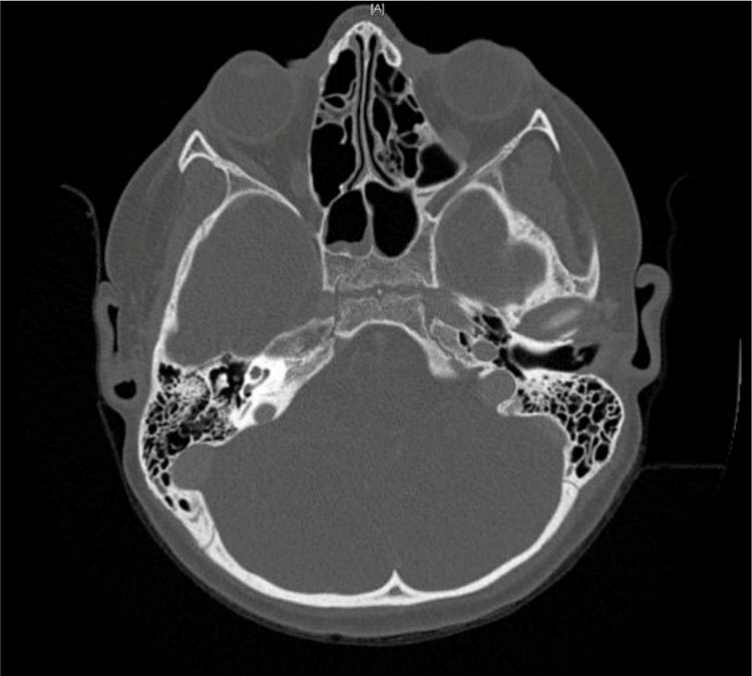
Axial CT taken 2 months after initial presentation demonstrates lucency of the right petrous apex representing partial pneumatization, as well as osseous erosion of the posterior cortex.
